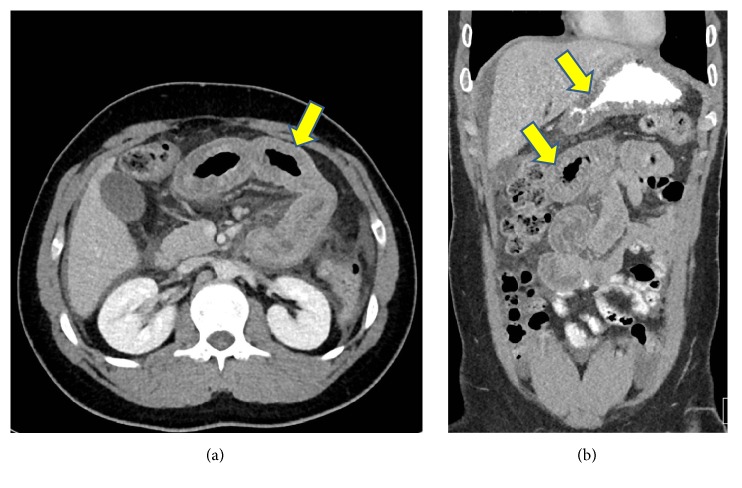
Abdominal CT scan during an acute attack showing long segments of enhancing mucosal thickening involving the proximal jejunum (arrows in a and b) and gastric mucosa (upper arrow in b).
